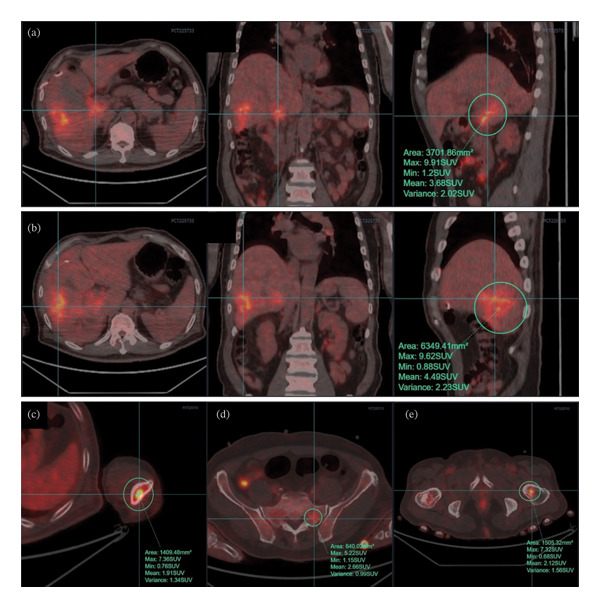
^18^F‐FDG PET/CT revealed hilar cholangiocarcinoma with intrahepatic and multiple osseous metastases. (a) The hilar cholangiocarcinoma demonstrated a standard uptake value (SUV) of 9.91. (b) The intrahepatic metastasis demonstrated an SUV of 9.62. (c–e) The multiple osseous metastases demonstrated SUVs ranging from 5.22 to 7.36.
